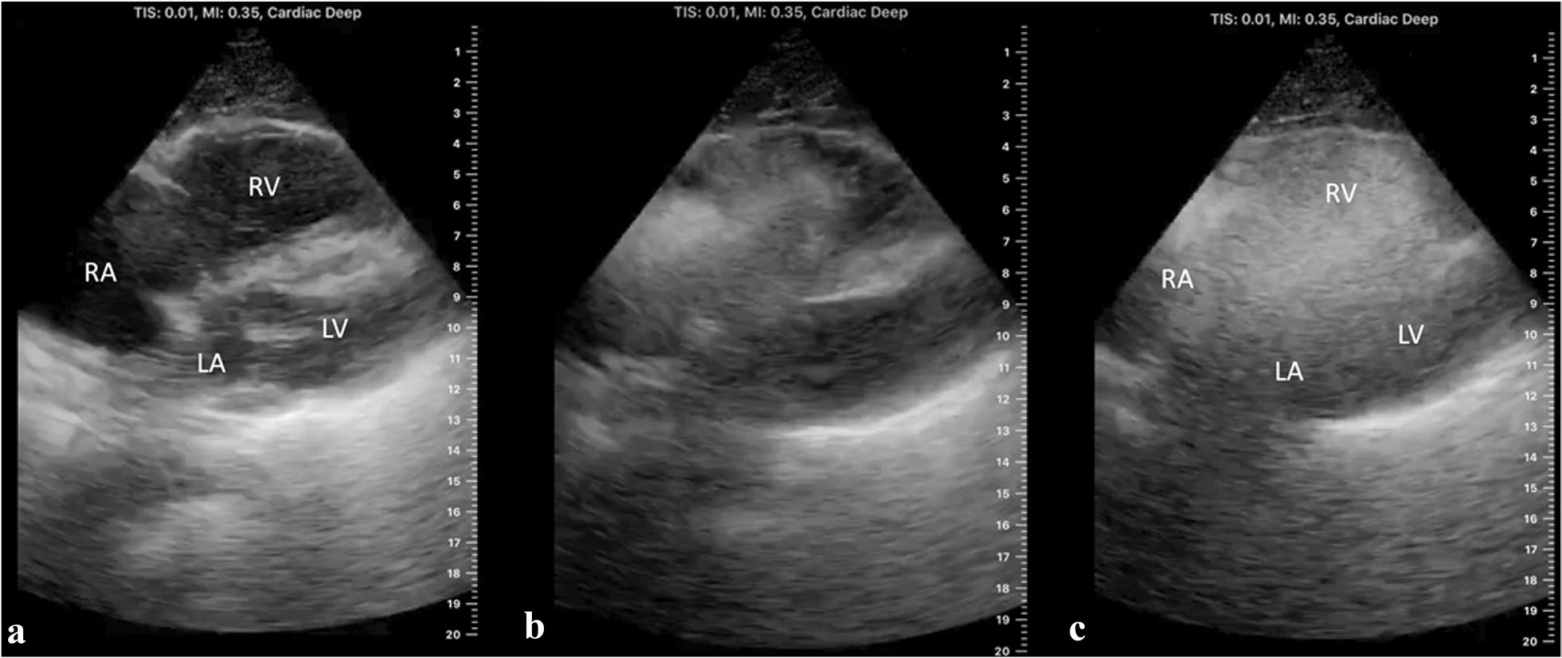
Interatrial shunt in a mechanically ventilated patient with acute cor pulmonale. Interatrial shunt in a mechanically ventilated patient with acute cor pulmonale due to COVID-19. a Labeled 2-D subcostal 4-chamber transthoracic echocardiographic (TTE) view of the heart demonstrating dilatation of the right atrium (RA) and right ventricle (RV). LA, left atrium; LV, left ventricle. b 2-D subcostal 4-chamber TTE view of the heart after i.v. injection of saline microbubble contrast showing the arrival of the contrast in the RA and RV. c Labeled 2-D subcostal 4-chamber TTE view of the heart after i.v. injection of saline microbubble contrast demonstrating grade 4 shunt (opacification of left atrium). Bubbles appeared in the LA within 3 cardiac cycles of opacification of the RA demonstrating the presence of an interatrial shunt
